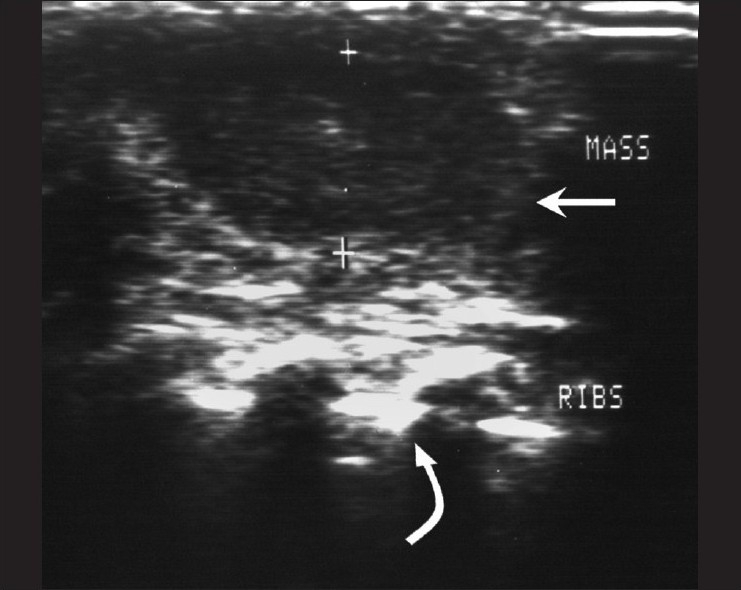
Rib tuberculosis – patient 7. Ultrasonography of the chest wall shows a hypoechoic collection with internal echoes (arrow) overlying the ribs (curved arrow)
